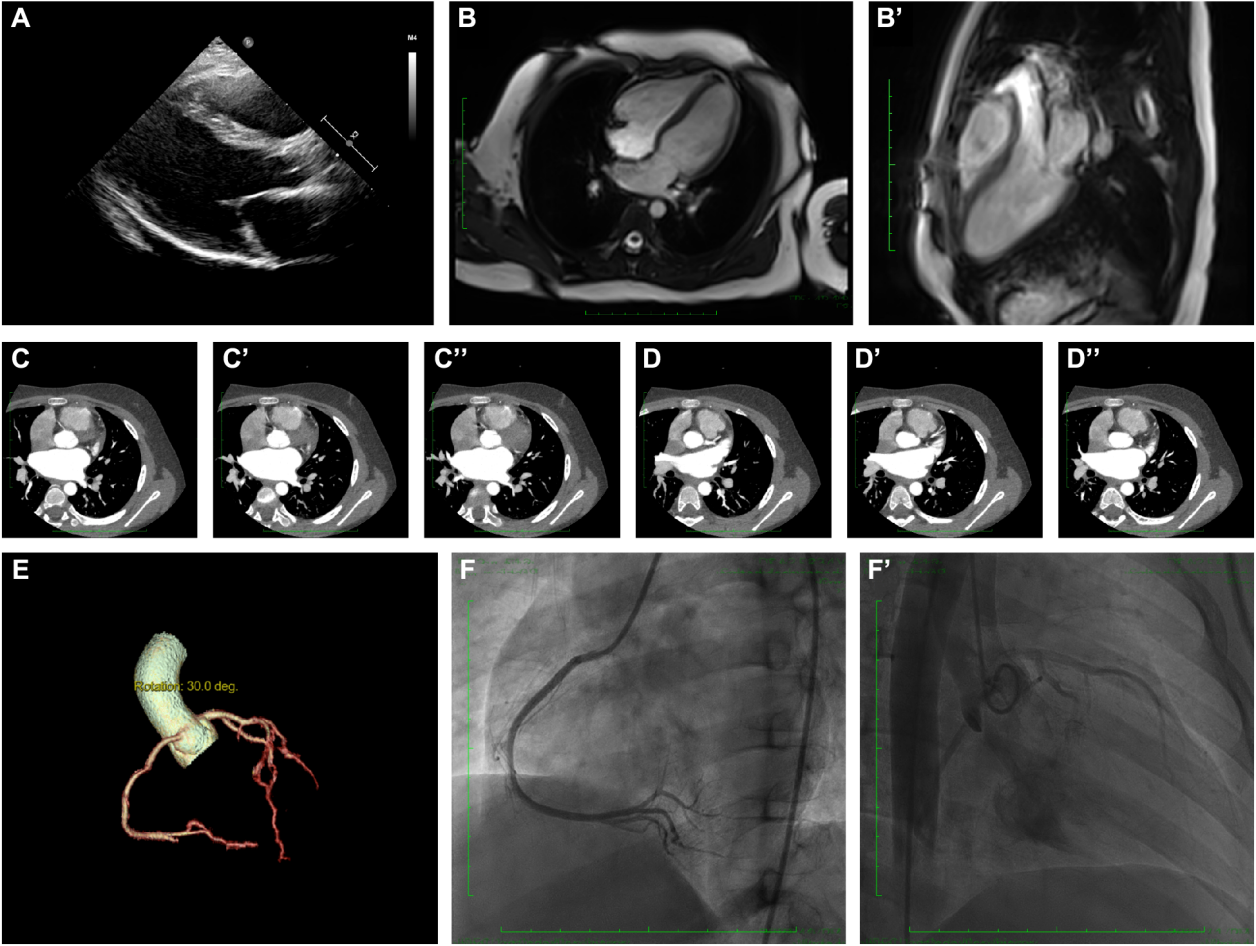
Clinical and radiographic manifestation of the current proband. (A) Echocardiography revealed a slight enlargement of the left ventricle. (B–B’). Cardiac magnetic resonance showed myocardial edema in the lateral ventricular wall and apex, indicating localized injures of the myocardium. (C–C”). CTA revealed normal right coronary artery structure. (D–D”) CTA revealed normal left coronary artery structure. (E) Coronary artery rebuilding based on CTA. (F–F’) Angiographic images of the left coronary artery; no significant positive result was recorded.
